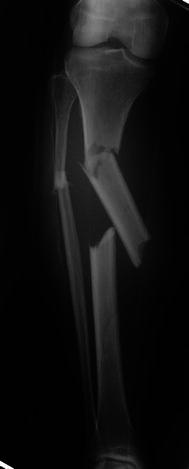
Preoperative radiograph of a segmental tibial fracture
